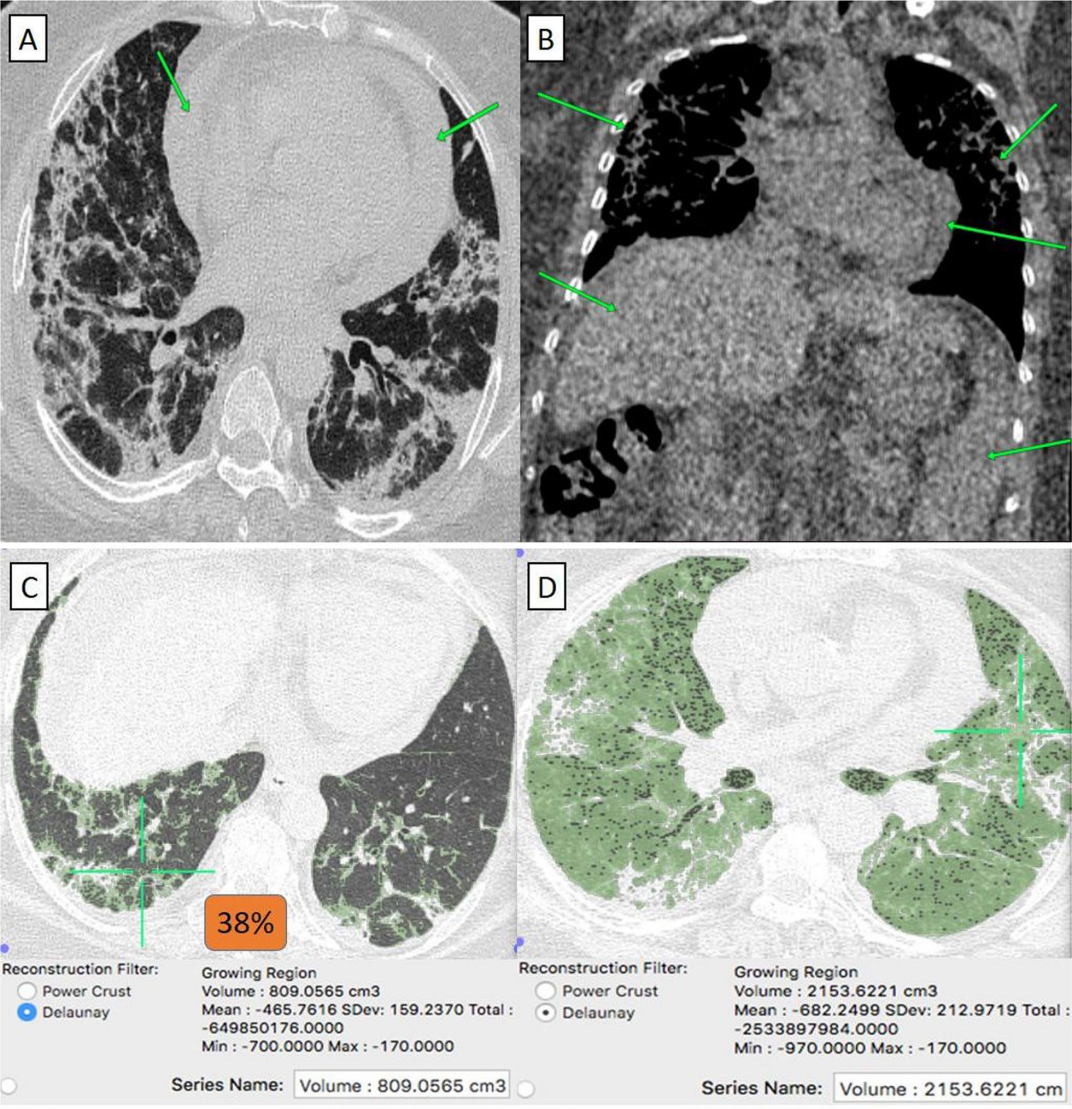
A 57-year-old female cardiac patient with history of HCV-related liver cirrhosis and had received “Oxford-AstraZeneca” vaccine then got COVID-19 infection. A Axial chest CT cut (lung window) showed bilateral mainly peripheral located heterogeneous ground-glass pneumonic patches with coarse septal thickening “patchy crazy-paving pattern” and large amount of pericardial collection (green arrows). B Coronal chest and upper abdomen CT cuts (mediastinal window) showed peripheral pneumonic patches, pericardial collection, liver cirrhosis and splenomegaly (arrows) C CT-Volumetry of the pathological lung parts averages 809 cc. [D] CT-Volumetry of total lung volume averages 2153 cc. So, 38% of the lung parenchyma was diseased (Score 2)
